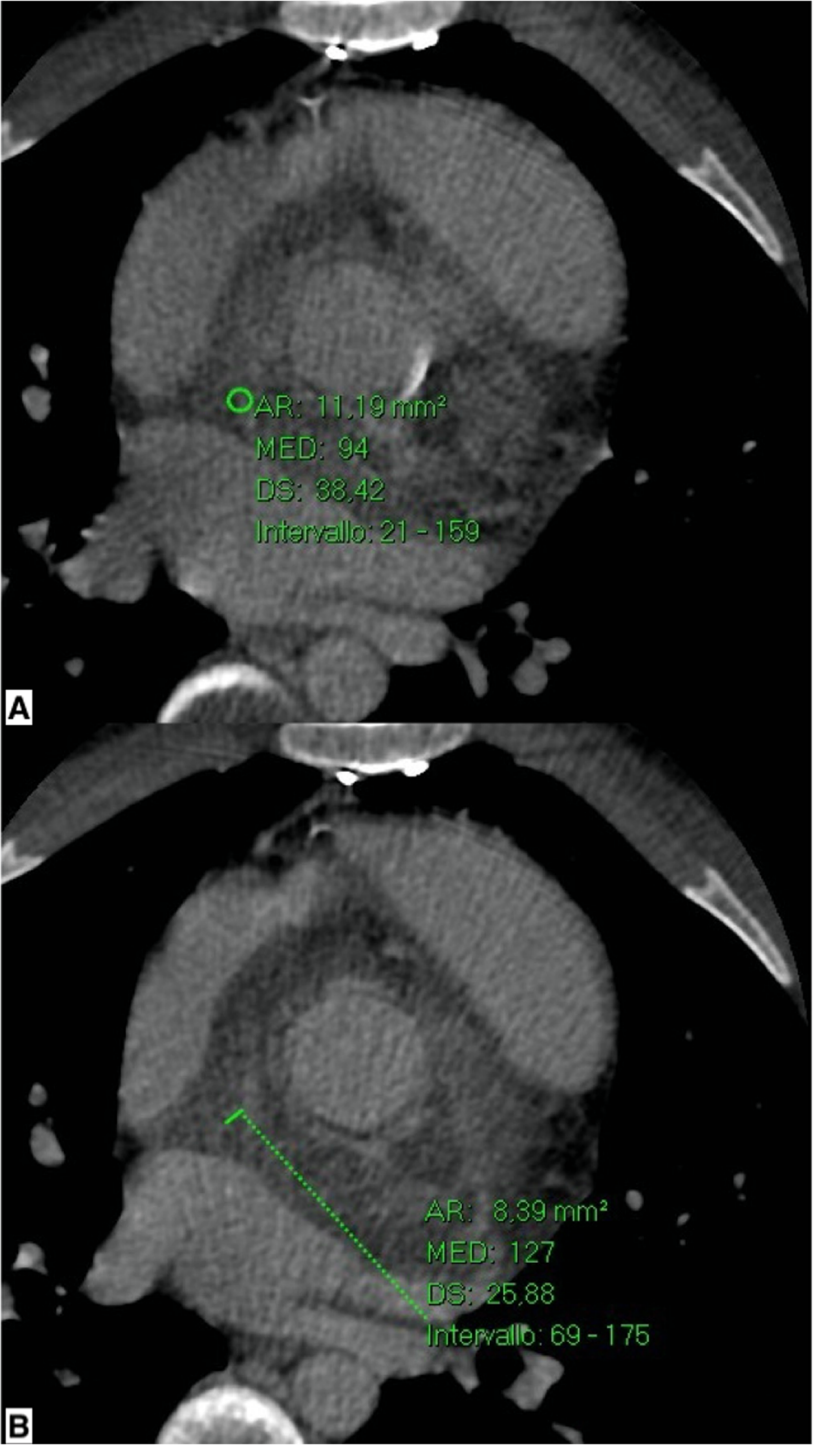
Computed tomographic scans. a Periaortic area shows maximum enhancement, 127 HU (Hounsfield Unit) in portal phase (70 seconds) with slow and progressive washout in late phase (b; 94 HU (Hounsfield Unit))
